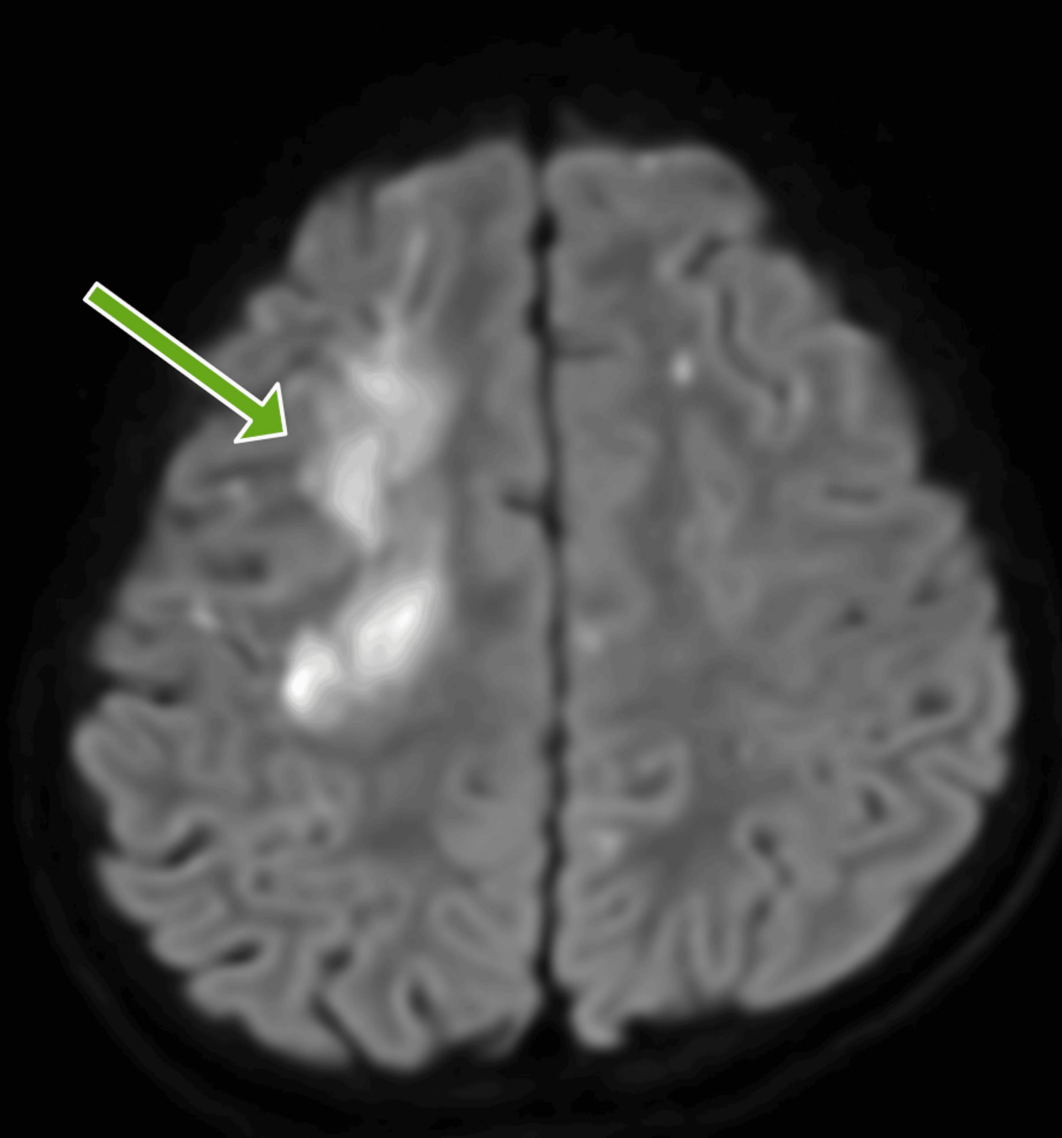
Diffusion-weighted imaging (DWI) showing acute multiple ischemic infarcts in the right frontoparietal area.
